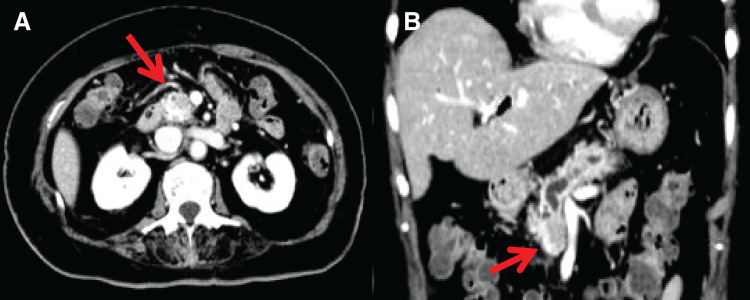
(A, B) Abdominal CT (pancreatic head). A hypovascular mass lesion was observed in the pancreatic head, partially in contact with the portal vein.
